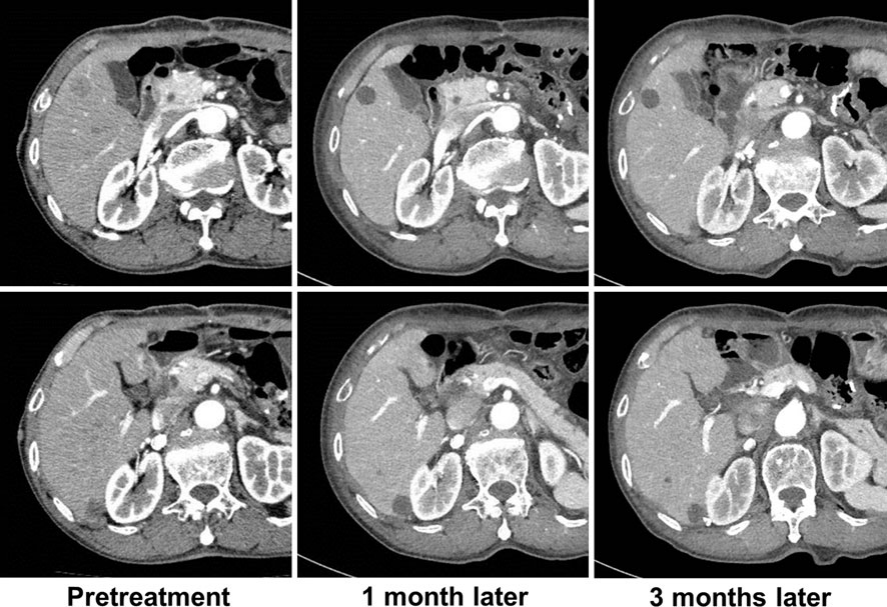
Contrast-enhanced computed tomography (CT) findings. Liver metastases were transformed into sharply circumscribed, non-enhanced homogeneous lesions 1 month after the start of imatinib therapy. CT conducted 3 months after the start of imatinib therapy showed that the response was still maintained. The *upper*
